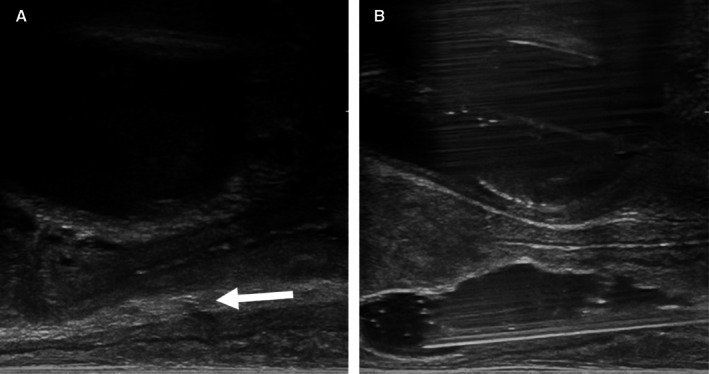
Transrectal ultrasound‐guided insertion of stabilised hyaluronic acid gel (sHA). (A) Identification of rectovaginal space (arrow). (B) Injection of sHA into the rectovaginal space.
